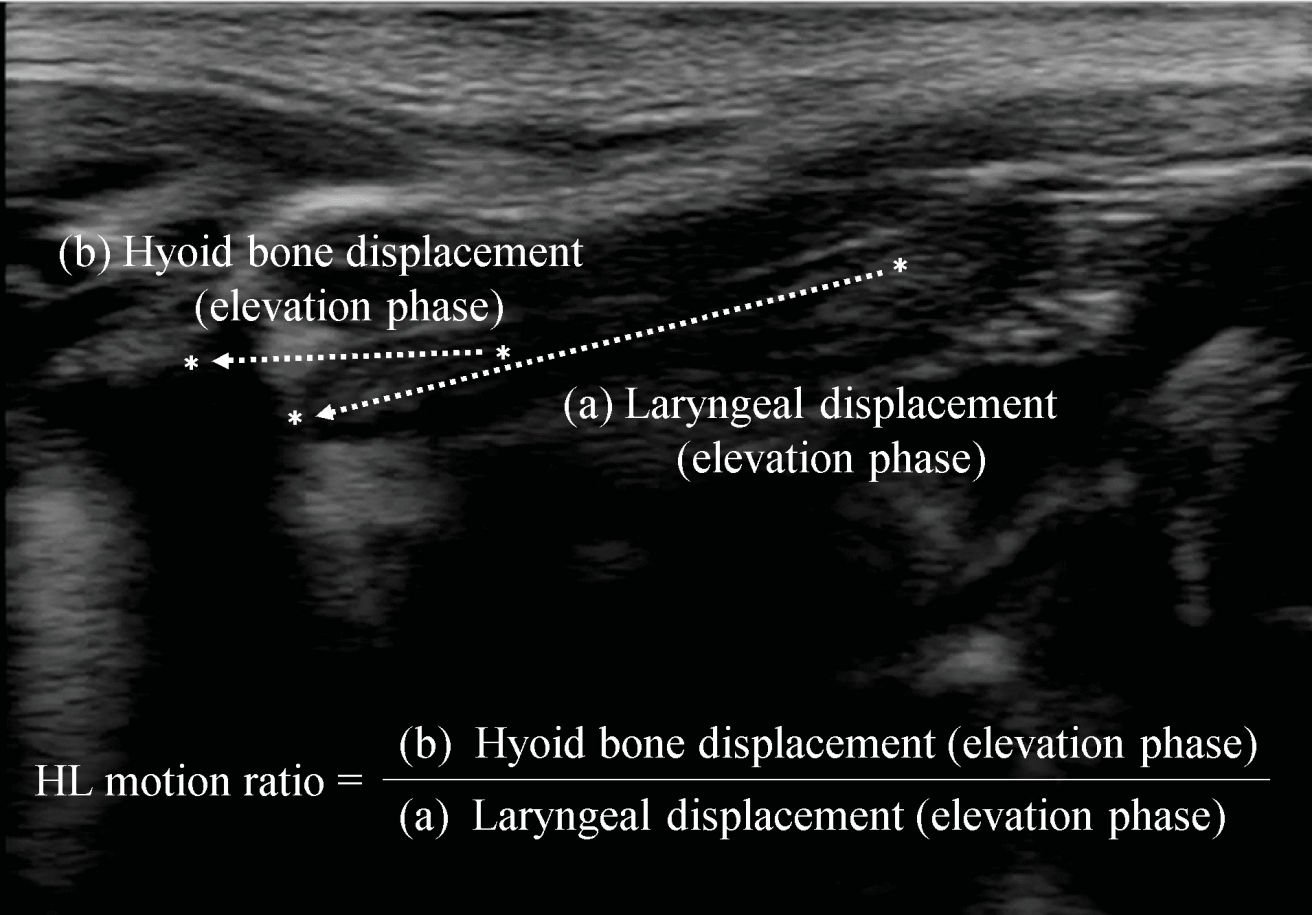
Visualization of the hyoid bone and larynx by ultrasonography, and the HL motion ratio. (A) Distance from the resting position at which elevation of the upper end of the thyroid cartilage reaches the maximally elevated position, at which elevation stops.(B) Distance from the resting position at which elevation of the hyoid bone reaches the maximally elevated position, at which elevation stops.
